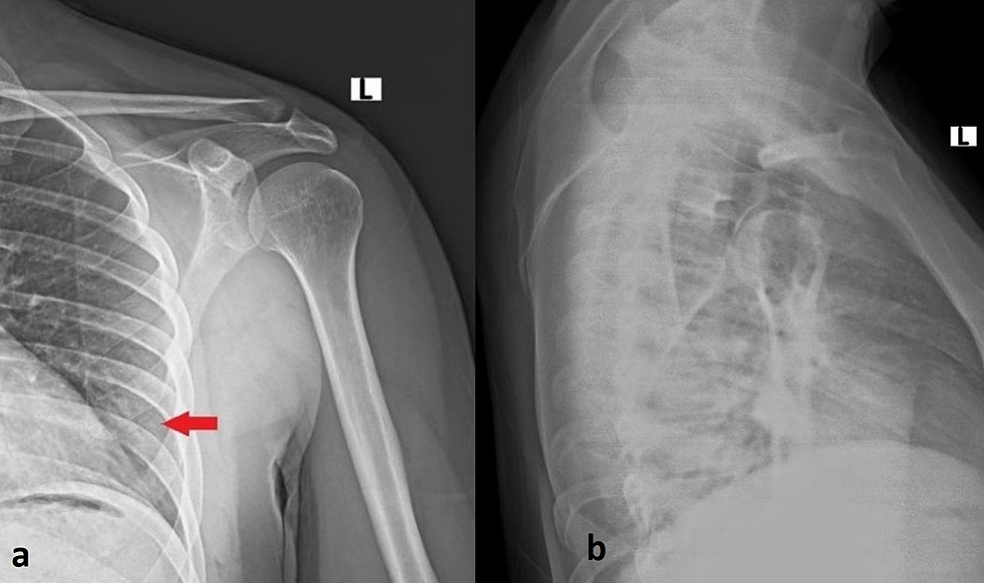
Anteroposterior (a) and transthoracic (b) shoulder x-rays did not reveal any fracture Red arrow depicts where the ISAF is located ISAF: inferior scapula angle fracture
